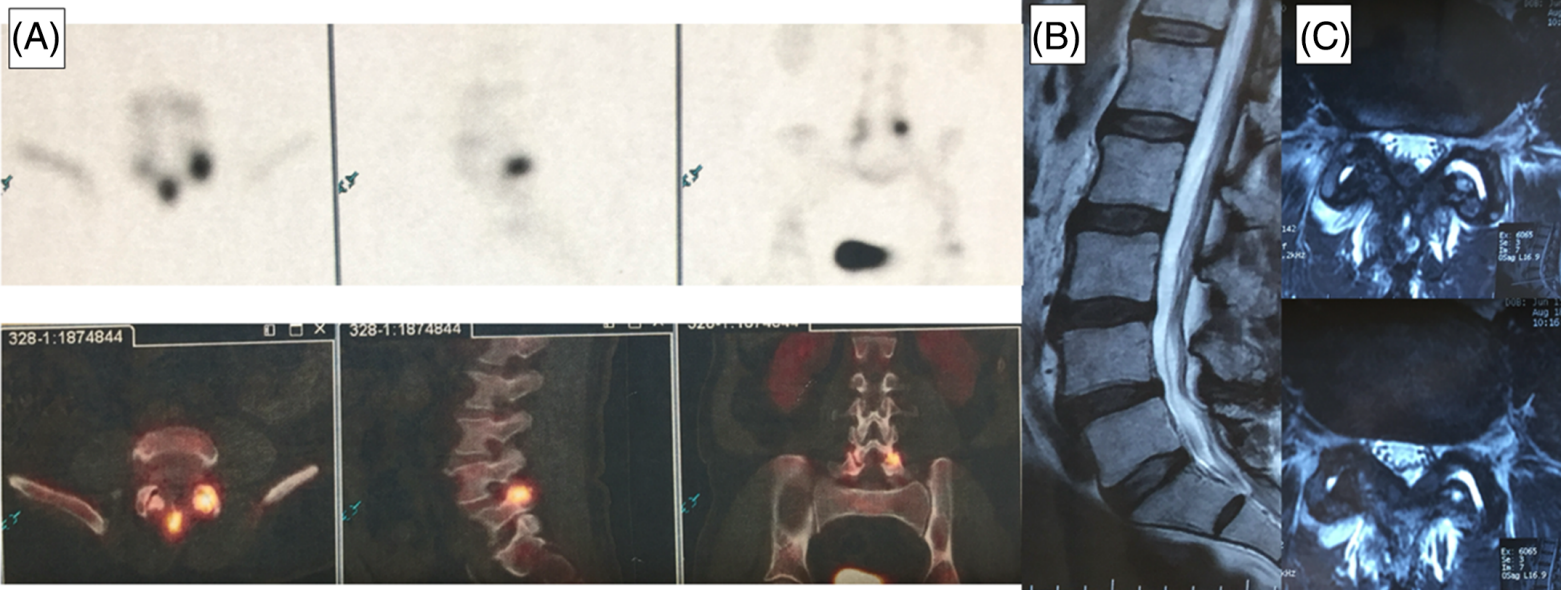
(A) Bone scan demonstrating uptake of Tc‐99 m on the left L4‐L5 facet joint. (B) Sagittal MRI demonstrating no evidence of disk bulge, neuronal compression, high‐intensity zones. (C) Axial MRI of the L4‐L5 level demonstrating a high‐intensity region of the left L4‐L5 facet joint.
